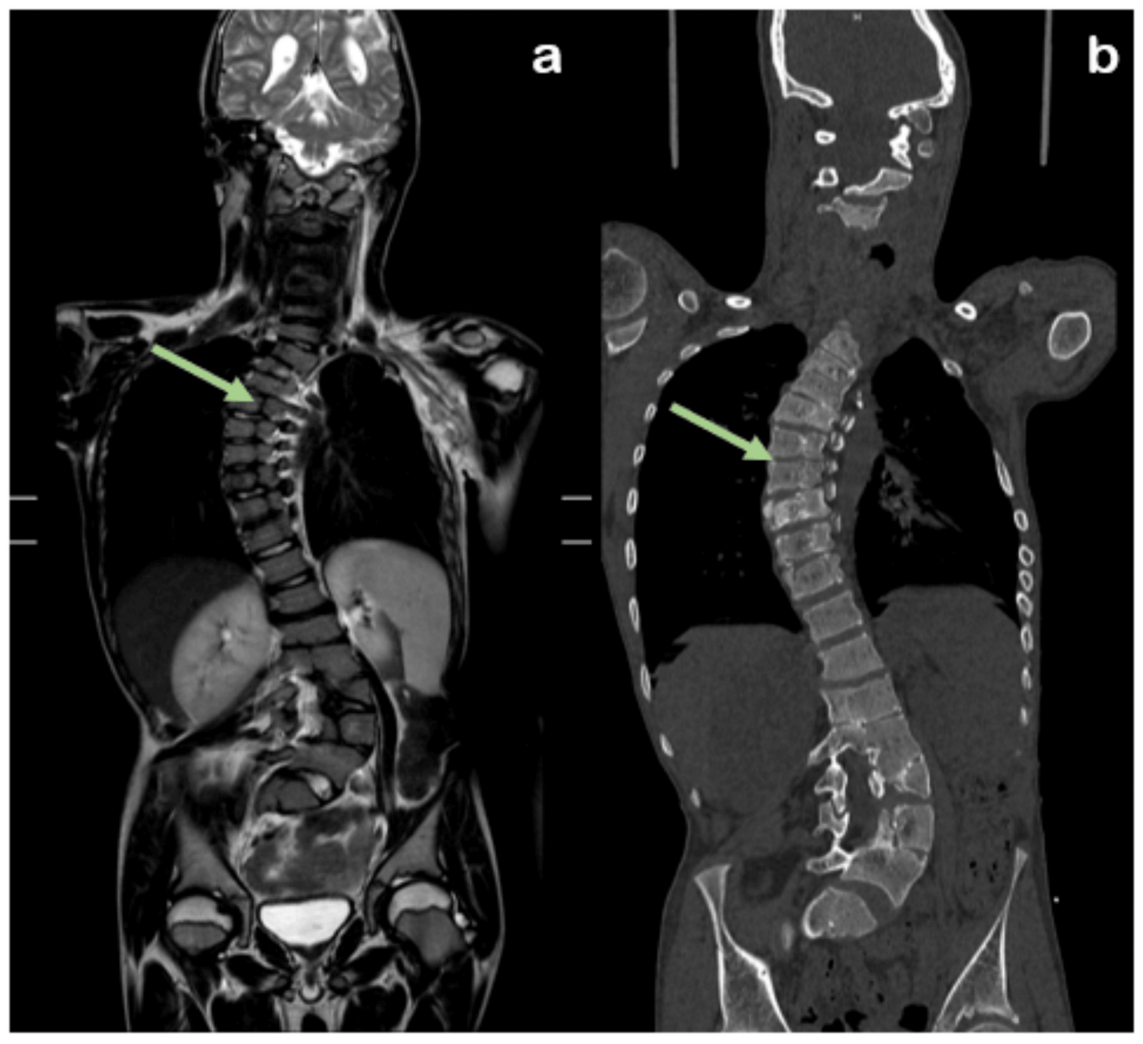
(a) Coronal section images of the spine from magnetic resonance imaging (T2 sequence) and (b) computed tomography scans reveal butterfly vertebrae (green arrow) extending from the D4 to L2 vertebral levels.
